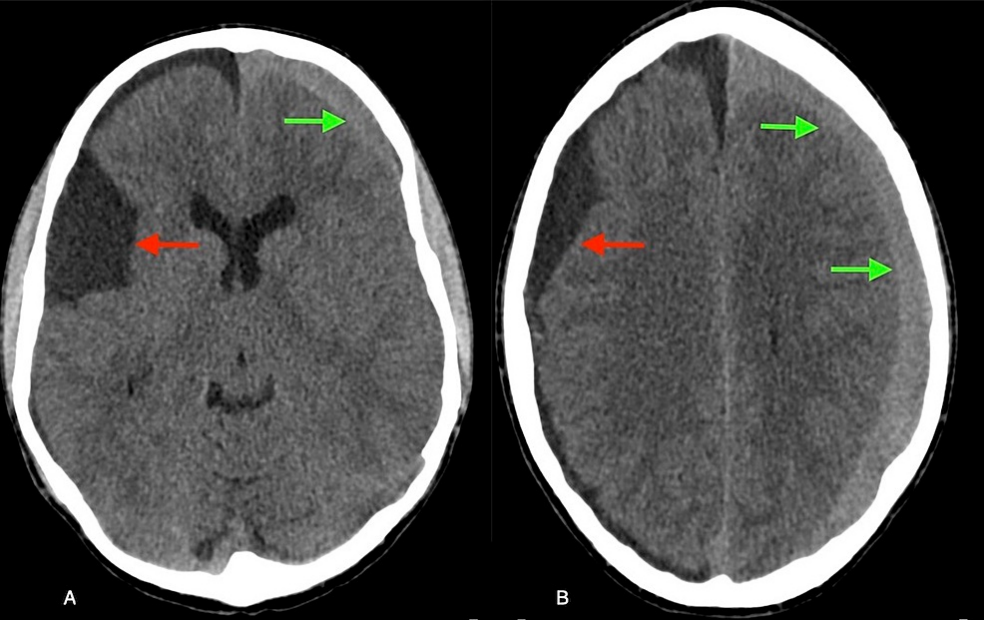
Axial CT scan of the head without contrast showing a new right chronic appearing subdural hematoma (SDH) (red arrows) and acute left SDH (green arrows).
